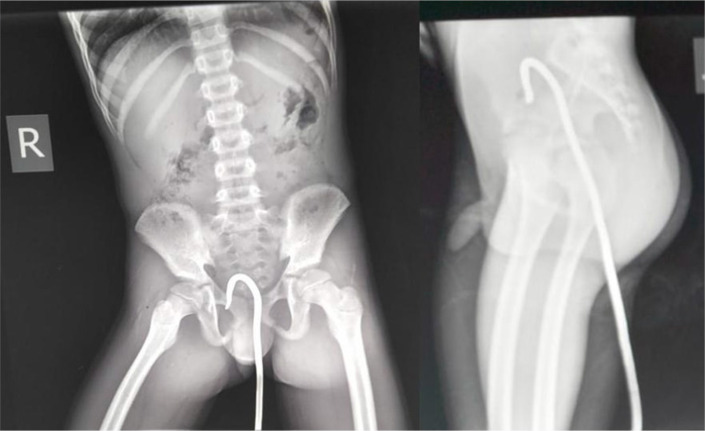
X-Ray Showing Metallic Object with Curved End into Rectum with No Emphysema
